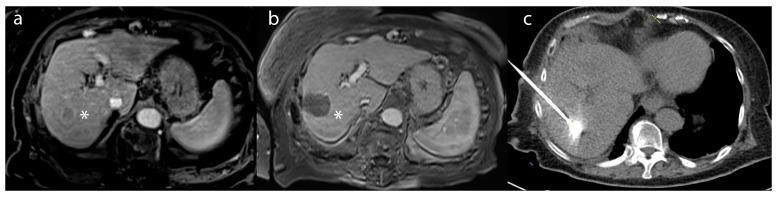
A 55-year-old male patient with (a) a lesion in segment 6 biopsy–proven as hepatocellular carcinoma (*)—on post-contrast T1-weighted imaging. (b) After microwave ablation, the lesion (*) demonstrated a lack of enhancement compatible with a complete radiographic response on the 1-month follow-up MRI (c) intraprocedural treatment CT of microwave ablation.
